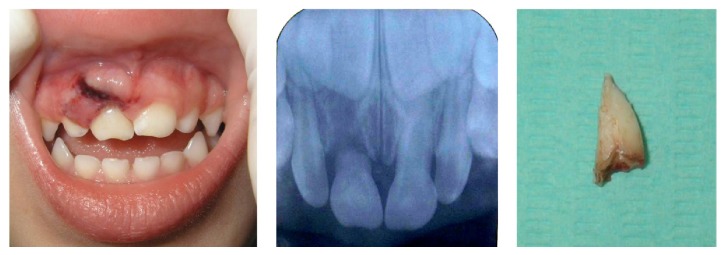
Intraoral view two hours after trauma. Periapical radiography. Root of the primary maxillary right central incisor root.
